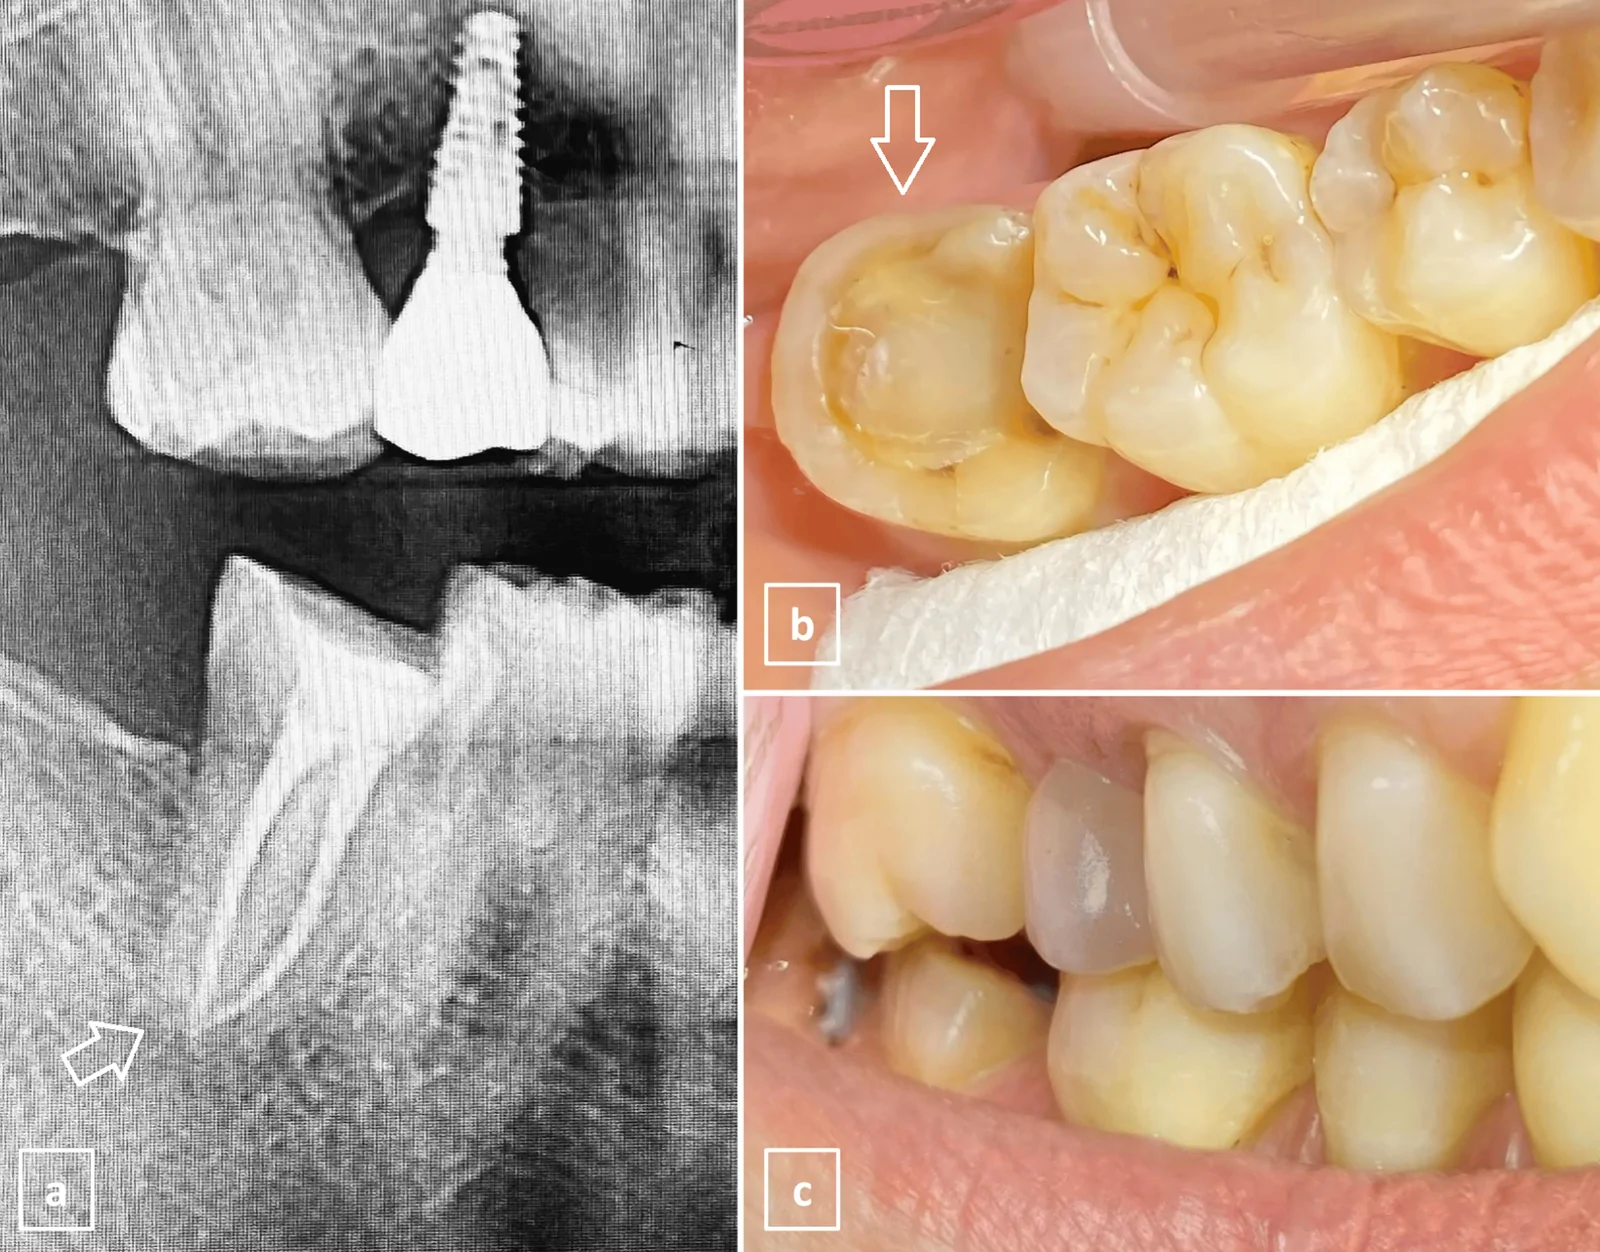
Initial clinical and radiographic examination (a) Preoperative radiograph showing the endodontically treated mandibular right second molar (tooth 47) with adequate root canal filling and absence of periapical pathology.
